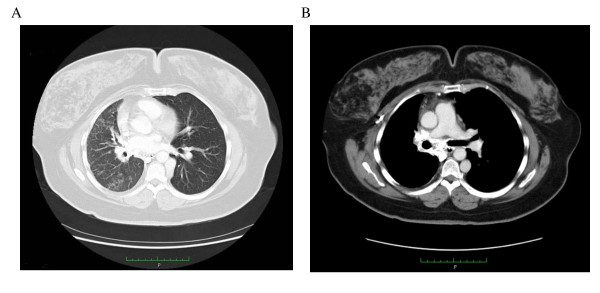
(A) Chest CT demonstrating volume loss of her right lung associated with increased interstitial markings and displacement of the mediastinum to the right. (B) A contrast-enhanced CT scan showed atresia of her right pulmonary artery.
